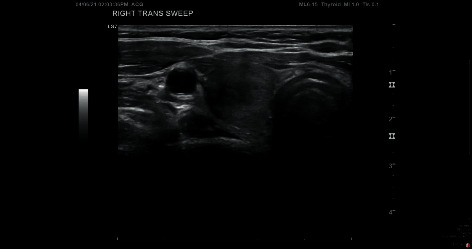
Ultrasound of the right thyroid lobe showing a heterogeneous mass.
